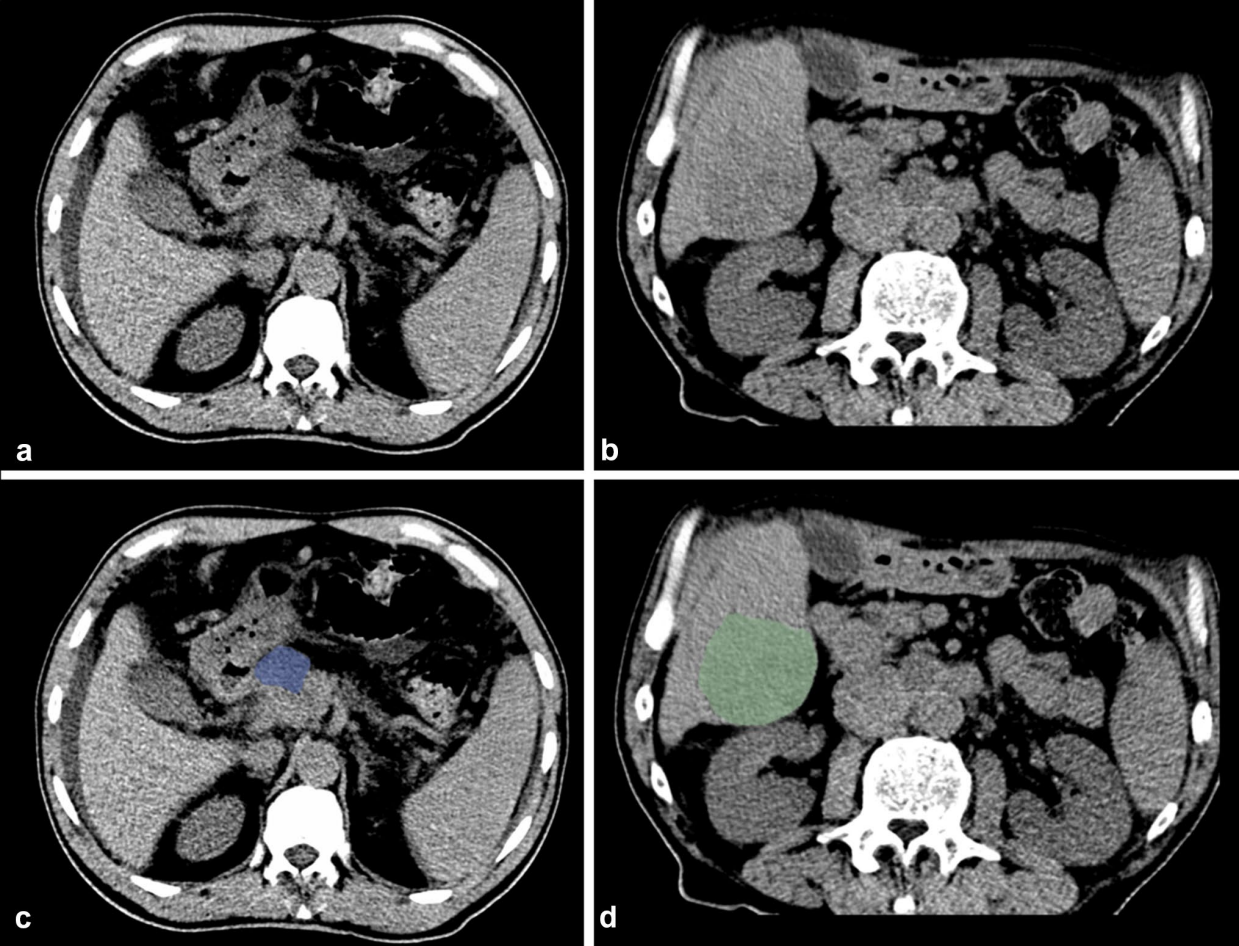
a–d 2D segmentation of primary PDAC (a, c) and hepatic metastasis of PDAC (b, d) on axial, non-enhanced CT. To prevent inclusion of any adjacent vessels or tissue, a small distance from the edge of the lesion was maintained
